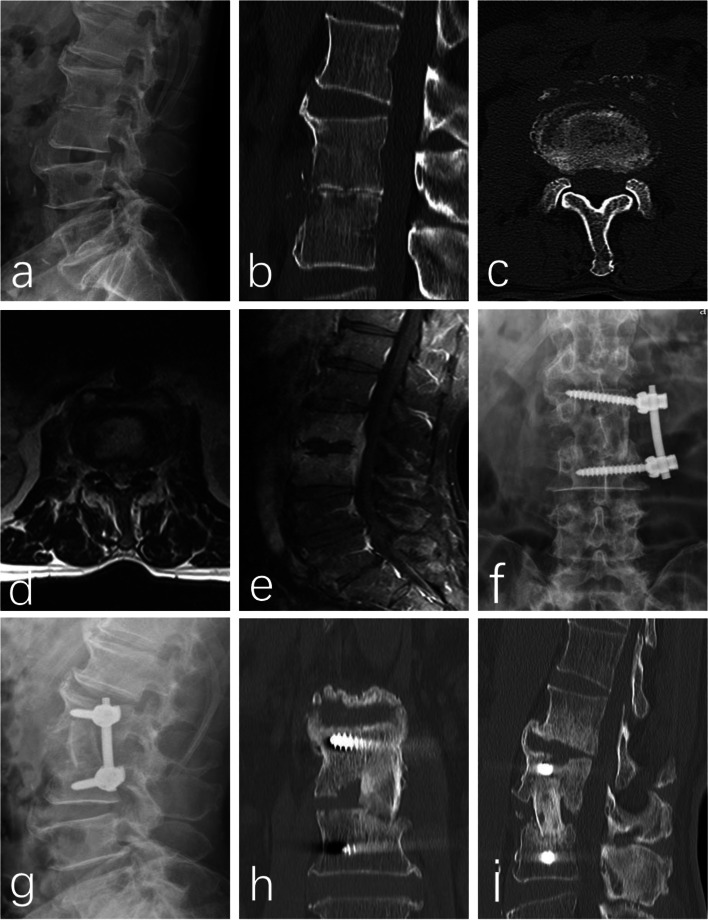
Sixty-five-year-old male with lumbar spondylodiscitis of L_2–3_. a Pre-op X-ray demonstrating disc space narrowing at L_2–3_. b, c Pre-op CT demonstrating bone destruction. d, e Pre-op MRI demonstrating abnormal signal, soft tissue swelling, and spinal cord compressed. f, g CT at 3 days after operation demonstrating Segmental lordosis and height was restored. h, i CT at 6 months follow-up demonstrating bony fusion
